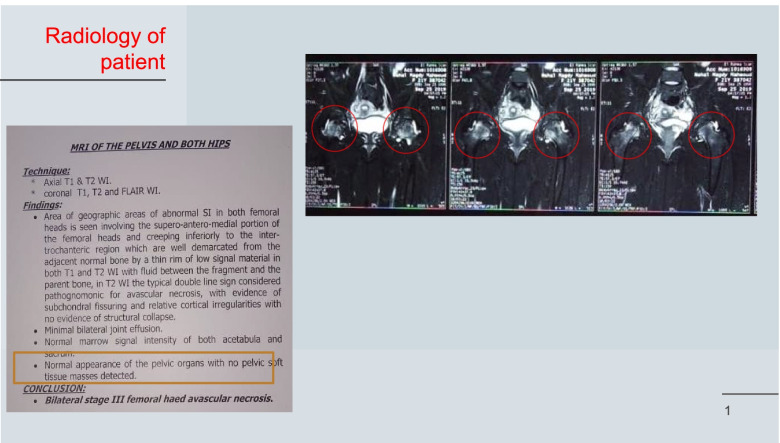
MRI of both hips revealed abnormal signals in the superior-antero-medial portion of both femoral heads and inferiorly to the intertrochanteric region which are well demarcated from the adjacent normal bone by a thin rim of low signal material in both T1 and T2 WI with fluid between the fragment and the bone. T2 WI revealed the typical double line sign which is considered pathognomonic for avascular necrosis with evidence of subchondral fissuring and relative cortical irregularities with no evidence of structural collapse. *Conclusion*: bilateral stage III femoral head avascular necrosis
